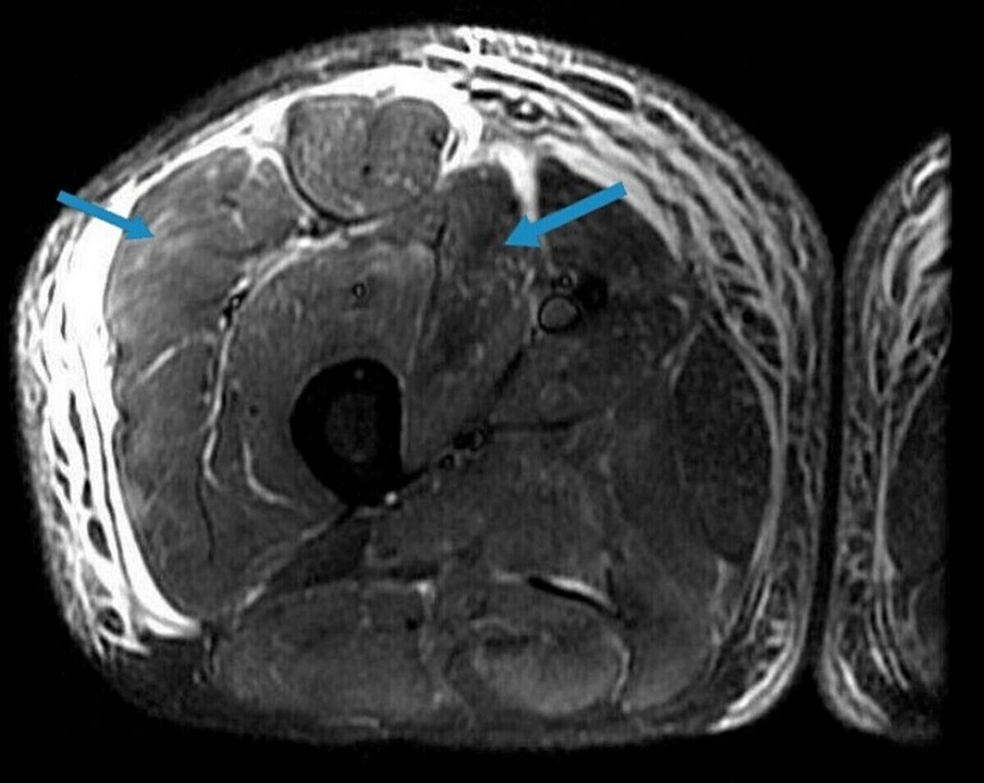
MRI of right thigh showing inflammatory changes (blue arrows) suggestive of myositis
